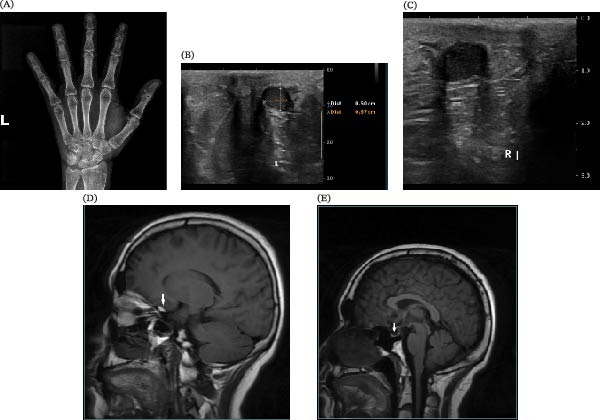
Bone age assessment, ultrasound, and magnetic resonance imaging (MRI) findings for the proband. (A) Digital radiography revealed complete epiphyseal closure. (B) Ultrasound imaging of the left testis revealed dimensions of 7 mm × 7 mm × 13 mm. (C) Ultrasound imaging of the right testis revealed dimensions of 6 mm × 7 mm × 12 mm. (D) The coronal brain MRI report demonstrated olfactory bulbs, revealed by white arrows. (E) Contrast‐enhanced MRI of the pituitary gland revealed a partially empty sella, as denoted by white arrows.
